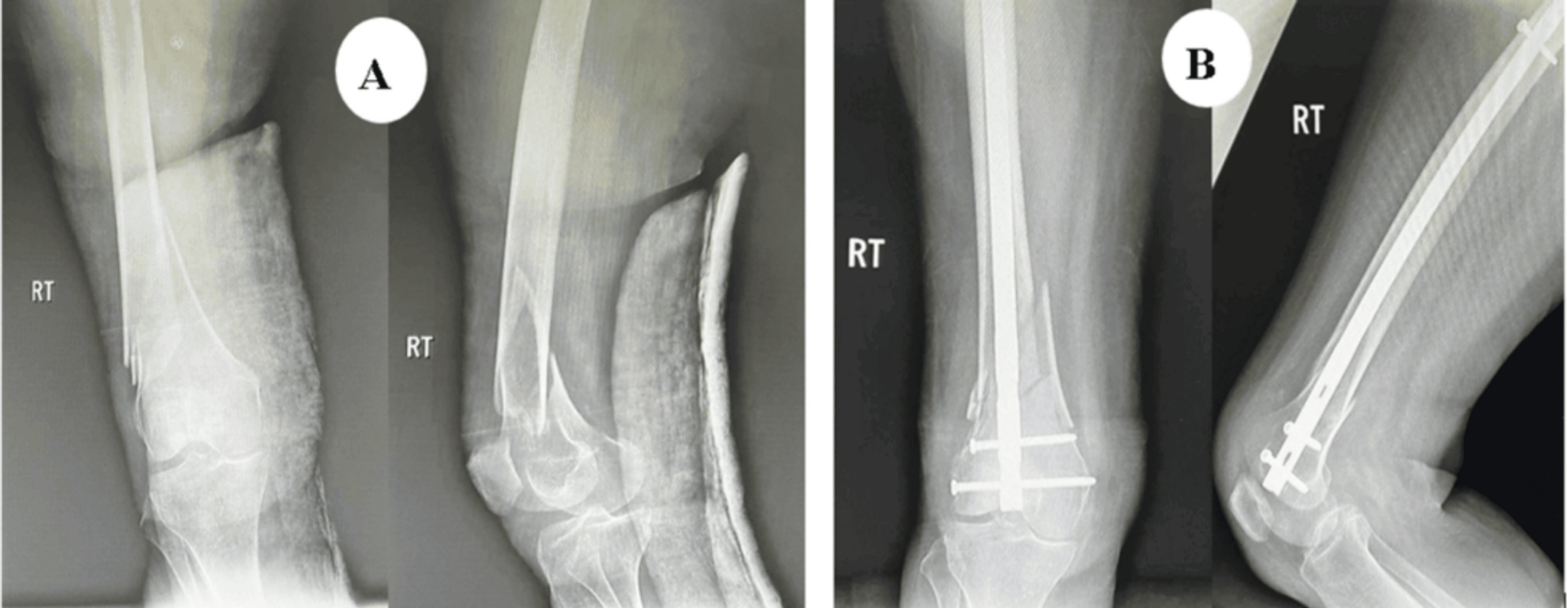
Case 6 A. preoperative anteroposterior and lateral X-rays, B. immediate postoperative X-ray.
